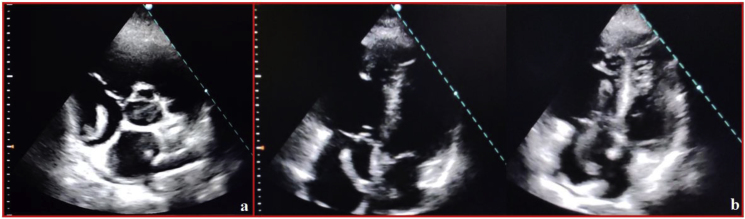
TTE para-sternal short axis view (a) and apical four-chamber view (b) showing a large thrombus inside the right cavities protruding into the left atrium.
